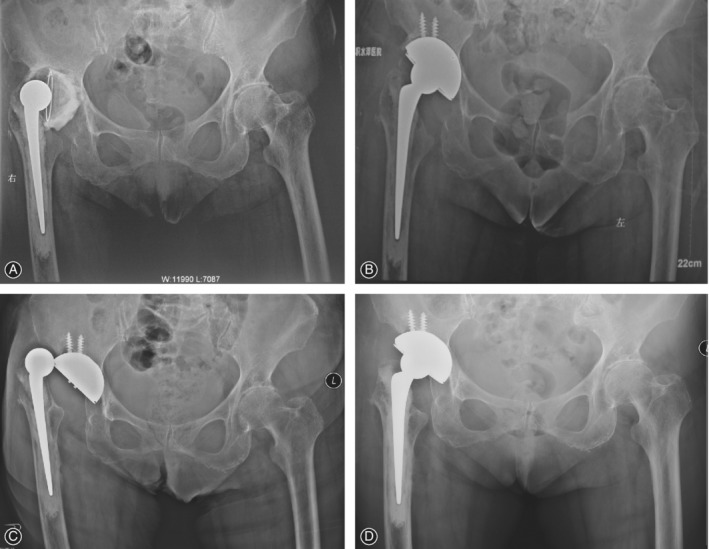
(A) Preoperative anteroposterior (AP) radiograph of the right hip in an 81‐year‐old woman requiring revision because of aseptic loosening. (B) AP radiograph right after revision arthroplasty with a 62‐mm diameter cementless acetabular component and a cemented stem. (C) The patient experienced recurrent dislocation 4.5 years later and was treated with closed reduction. (D) The components were well‐fixed at the 8.5‐year follow up.
